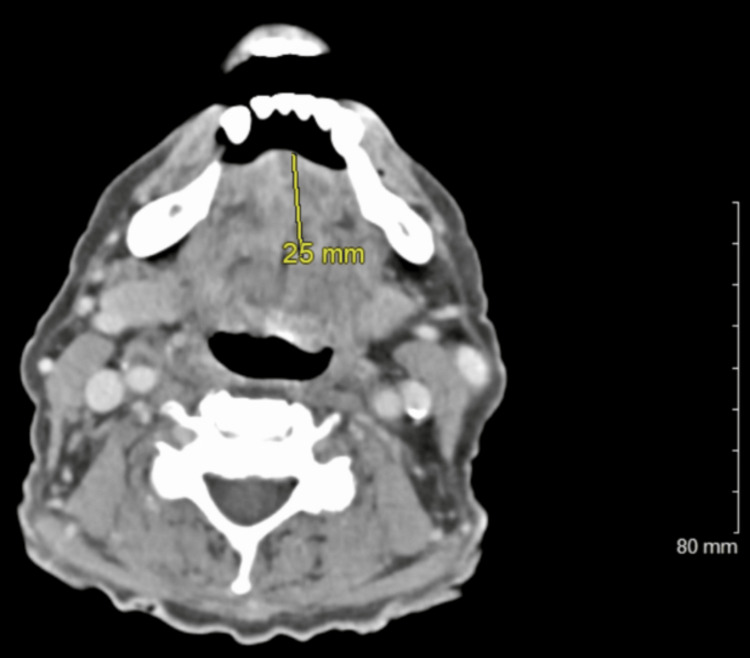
CT soft tissue neck, revealing a 2.5 cm wide heterogenous, necrotic, or cystic enhancement of the anterior tongue CT: computed tomography
